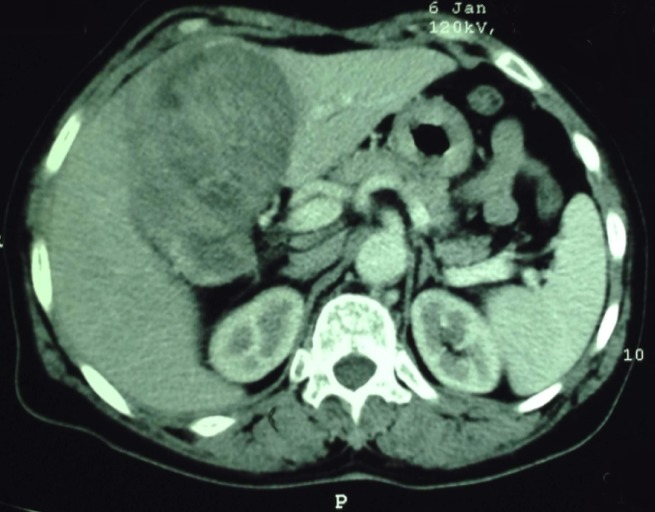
Abdominal CT: heterogeneous hepatic masse misdiagnosed as GIST
